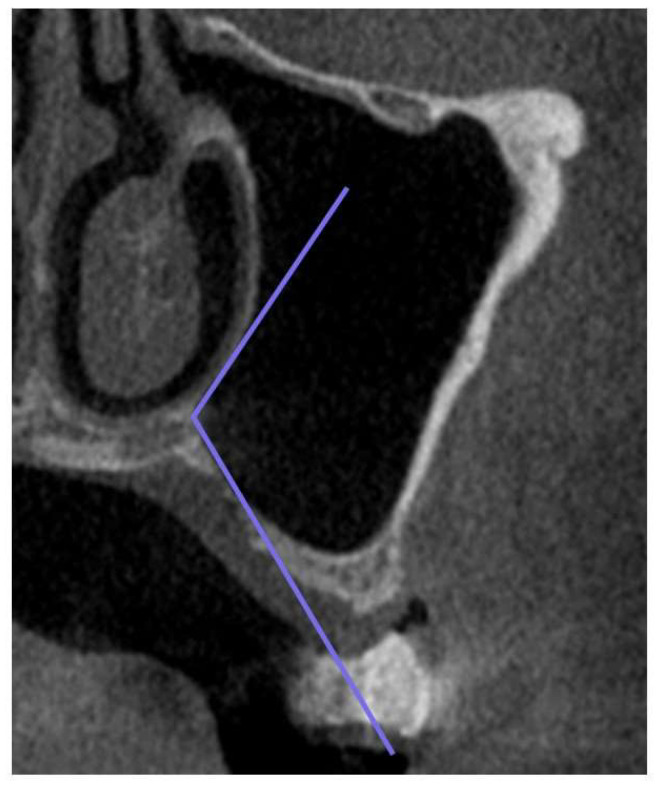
Palatonasal recess (PNR), between the roof of the hard palate and the lateral wall of the nasal cavity (purple line). Measurements were acquired within a plane perpendicular to the maxillary buccal bone of the tooth defect in the CT image.
